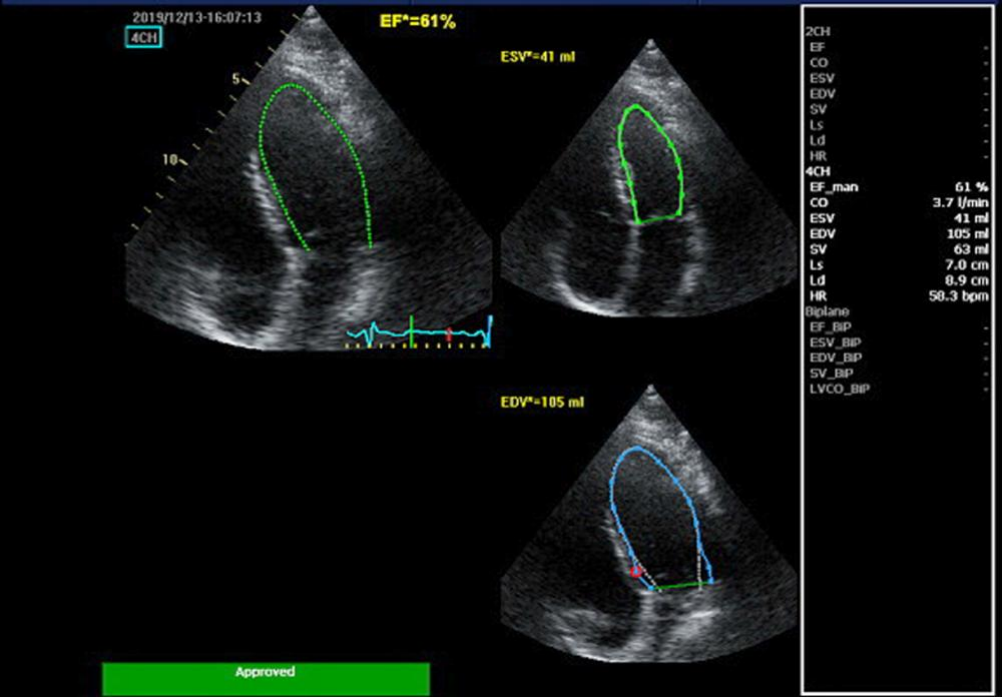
Follow-up transthoracic echocardiogram demonstrating consistently recovered left ventricular function, with a Simpson's biplane ejection fraction of 61%.
